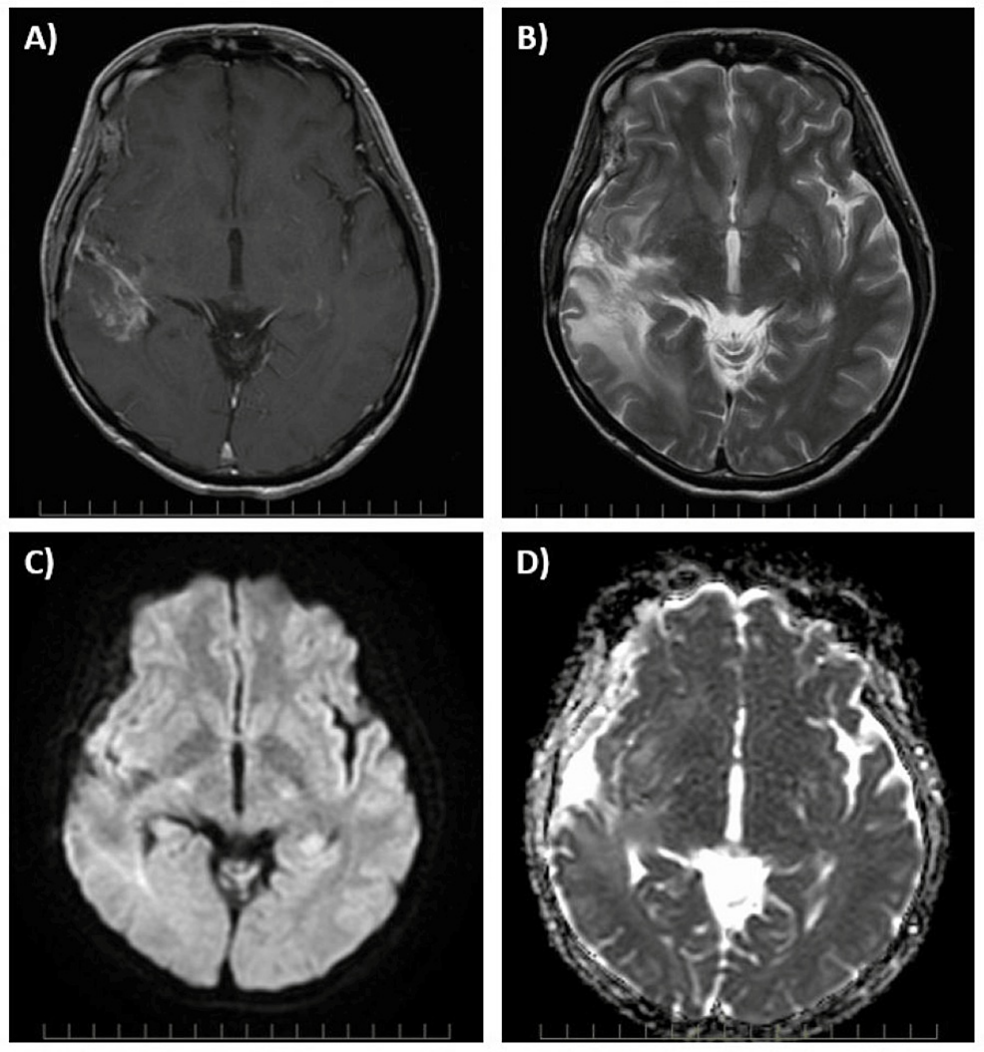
MRI brain findings at pseudoprogression six months following temozolomide plus TTFields therapy (Case 1) (A) The patient developed a new heterogeneously enhancing mass lesion in right temporal lobe on a contrast T1-weighted image; (B) T2-weighted image shows marked enlargement of brain edema; There was no diffusion restriction on diffusion-weighted image (C) or apparent diffusion coefficient map (D). TTFields: tumor-treating fields
